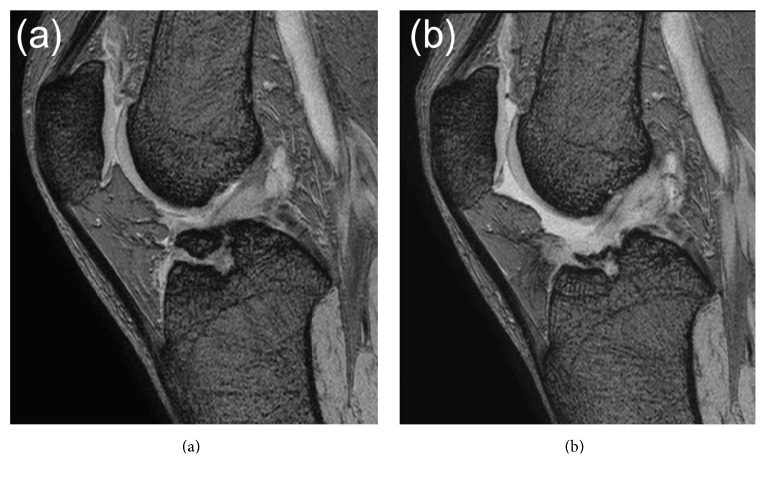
(a) Preoperative magnetic resonance imaging (MRI), showing no rupture in ACL fibers. (b) MRI 6 months after surgery showing only partial rupture of ACL fibers at the site of debridement.
